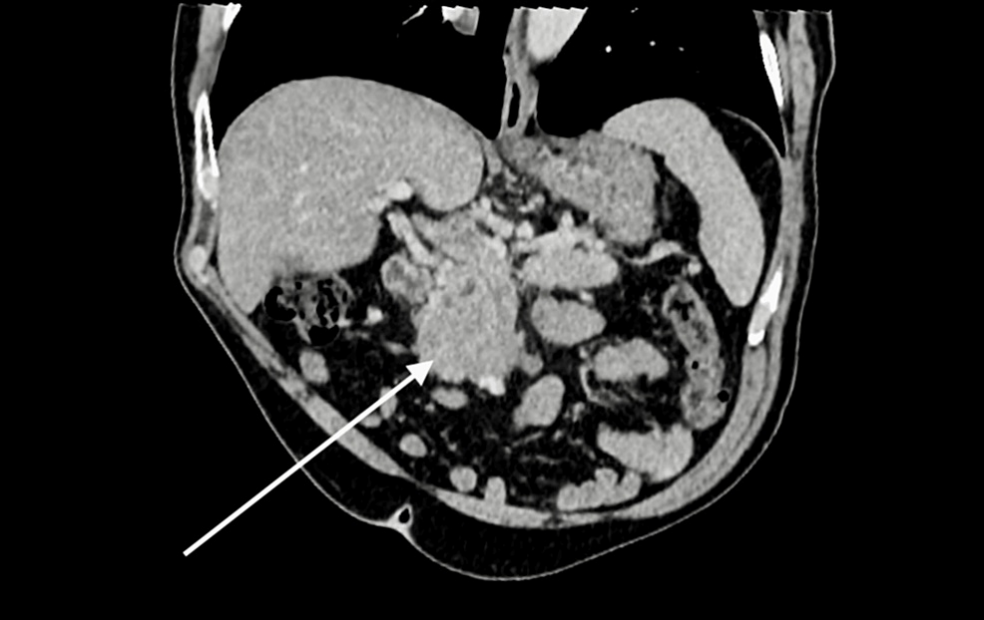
Contrast-enhanced reformatted abdominopelvic Computerized Tomography (CT) scan demonstrates interval growth of a pancreatic head soft tissue mass after two years.
